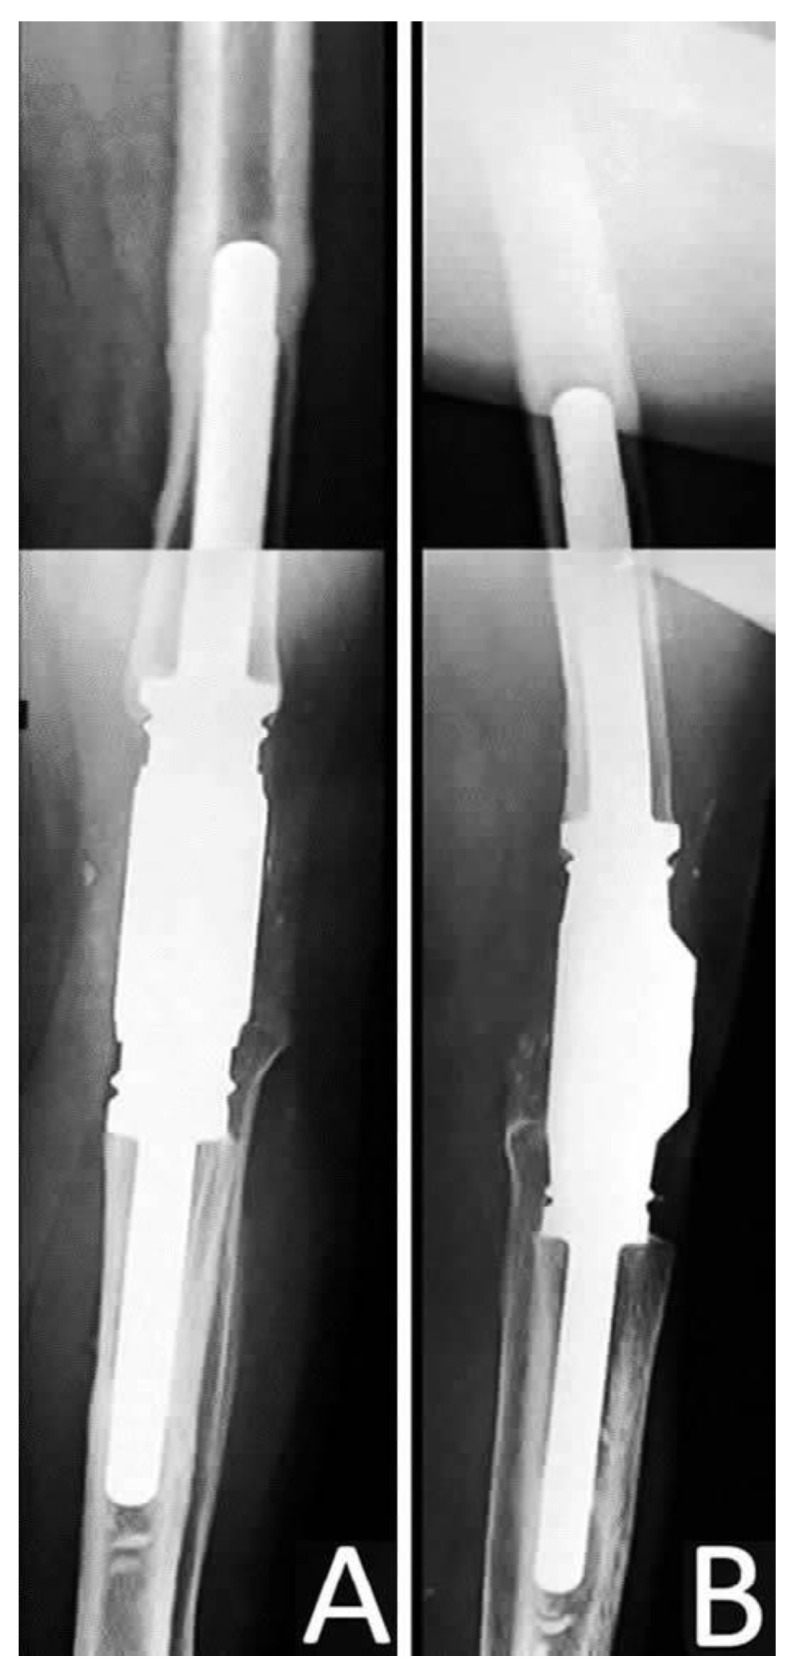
(A) Anteroposterior and (B) Lateral post-operative radiograph of the knee after the application of the silver-coated endoprosthesis.
